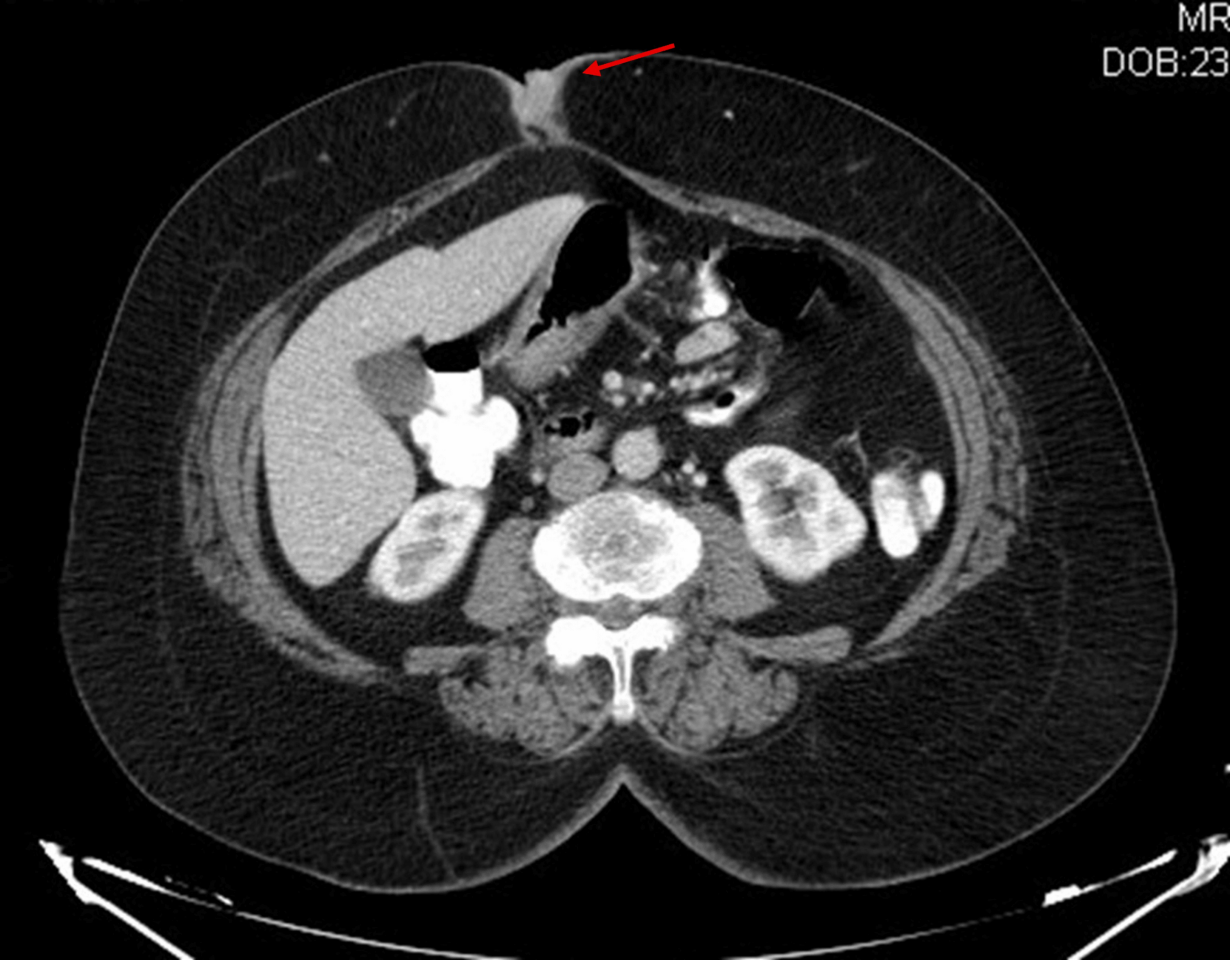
CT scan of the abdomen with contrast (axial view), showing the umbilical mass
